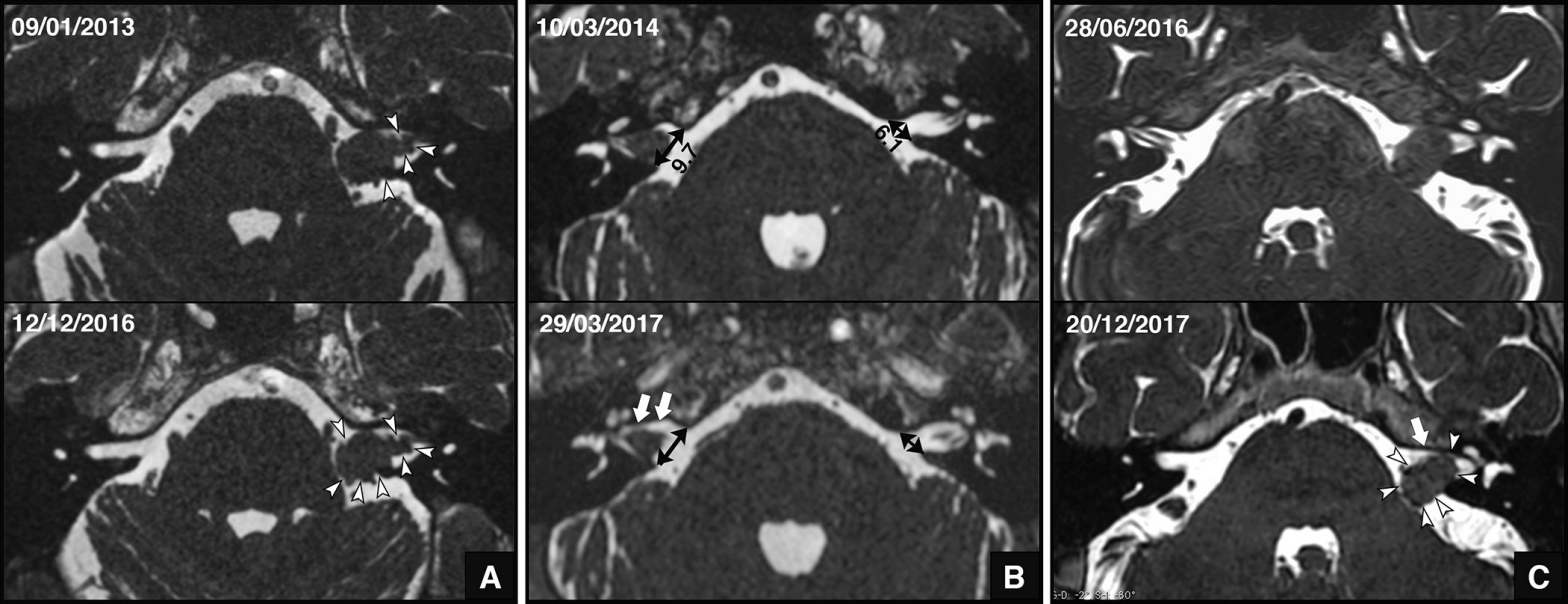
MRI aspect of shrinking tumors (HRT2-weighted imaging). A) First and last MRI of patient 5 that shows the festooned appearance (arrowheads). B) First and last MRI of patient 7 that shows the appearance of CSF infilling the IAM and the asymmetrical size of IAM (large arrows). Measurements are in mm and did not change between the first and last MRI. C) First and last MRI of patient 13: arrows indicate the festooned appearance and large arrows indicate CSF infilling the IAM.
